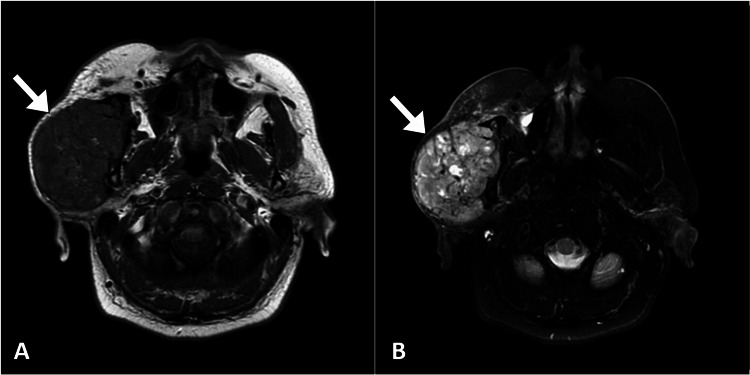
Axial T1-weighted (A) and T2-weighted (B) MR images show a heterogeneous mass (arrow) in the right parotid gland. MR: magnetic resonance
